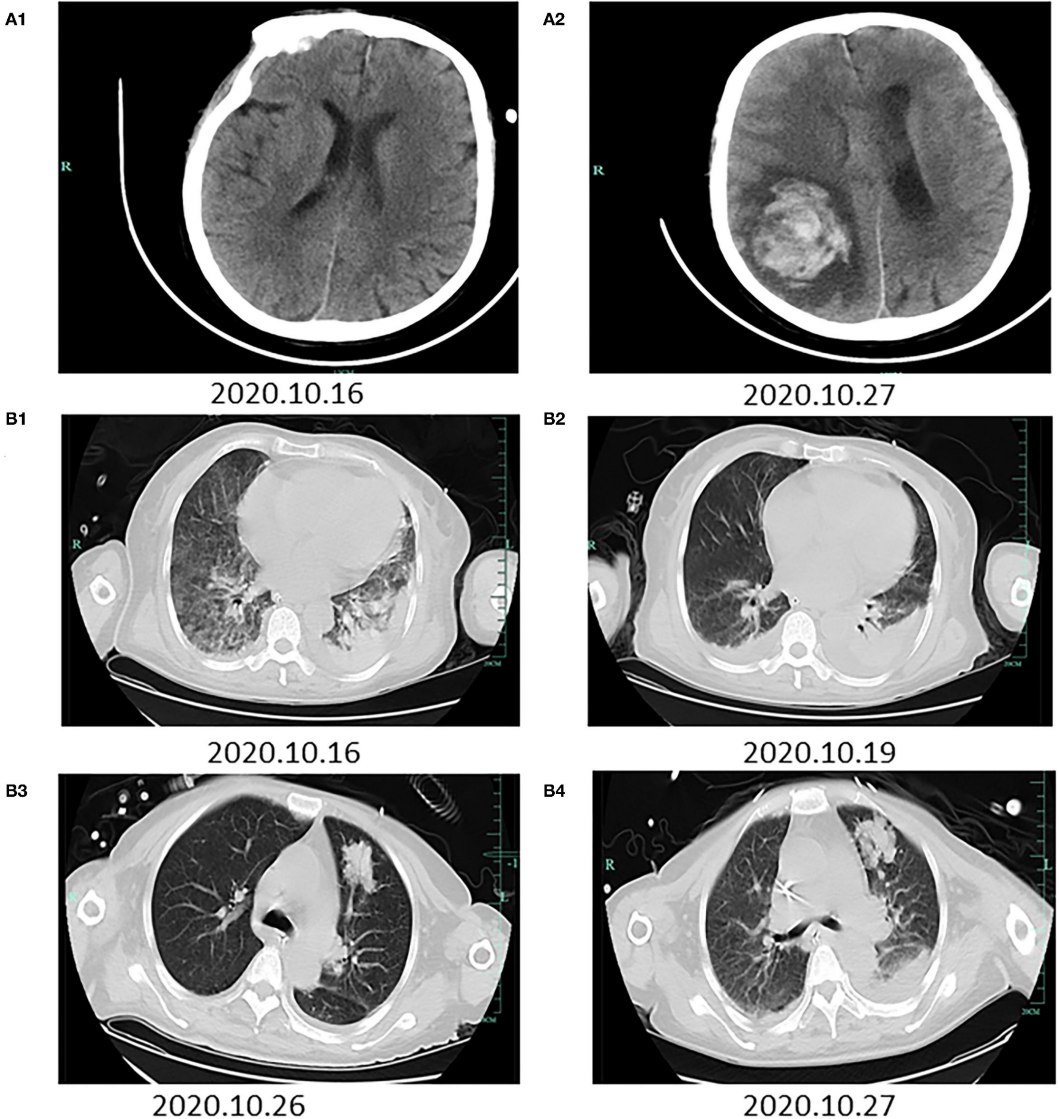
Radiographic images during the hospitalization. The first row of brain CTs (A1,A2) was taken on October 16 and 27, 2020, respectively. (A1) A normal brain. (A2) Hemorrhage in the right paraoccipital lobe that had ruptured the lateral ventricle. The second and third row of chest CTs (B1–B4) was taken on October 16, 19, 26, and 27, 2020, respectively. (B1) Bilateral patchy shadows and diffuse infiltration and extensive consolidation in the right lung. (B2) Hydrothorax and relief of bilateral exudation. (B3) New patchy shadows in the right upper lung. (B4) Patchy shadows in the right upper lung and bilateral exudation.
